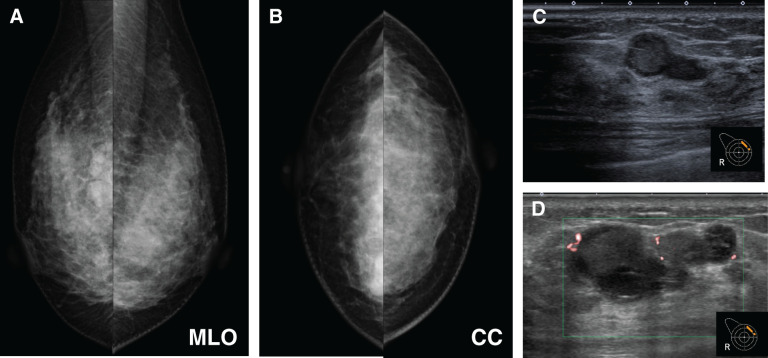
Mammography performed at the referring clinic in March, year X, prior to core needle biopsy, showed focal asymmetric density in the middle to inner region of the right breast (A, B). US performed at the referring clinic in March, year X, prior to core needle biopsy, demonstrated a 5-mm hypoechoic mass with mixed echogenicity in the upper inner quadrant of the right breast (C). US performed at our institution after core needle biopsy demonstrated hematoma-like changes associated with disruption of the cyst wall. The green box indicates the color Doppler sampling box (region of interest), which defines the area evaluated for vascularity using color flow imaging (D). CC, cranio-caudal; MLO, medio-lateral oblique
